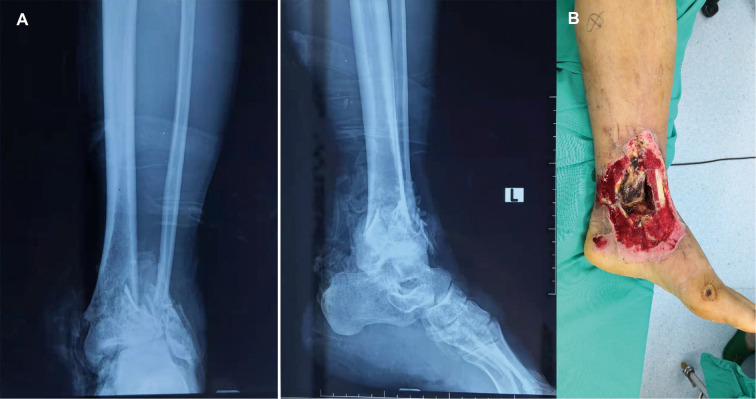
(A) A 55-year-old man sustained injury due to a road traffic accident that resulted in a Gustilo grade IIIB fracture in the left medial ankle. Anteroposterior and lateral X-ray radiographs show a tibial and fibula bone fracture. (B) Soft tissue defects in the medial ankle and distal tibia shows sign of infection and necrosis.
